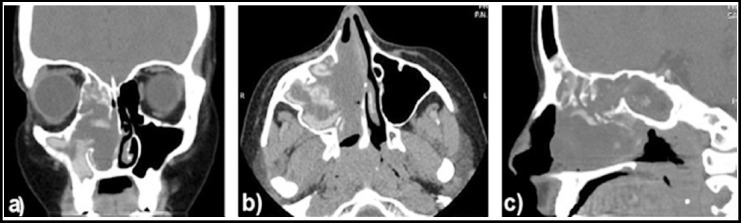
Computed tomography images of adult female patient revealed multiple polyps in the right paranasal sinuses a) coronal section, b) axial section, and c) sagittal section.
